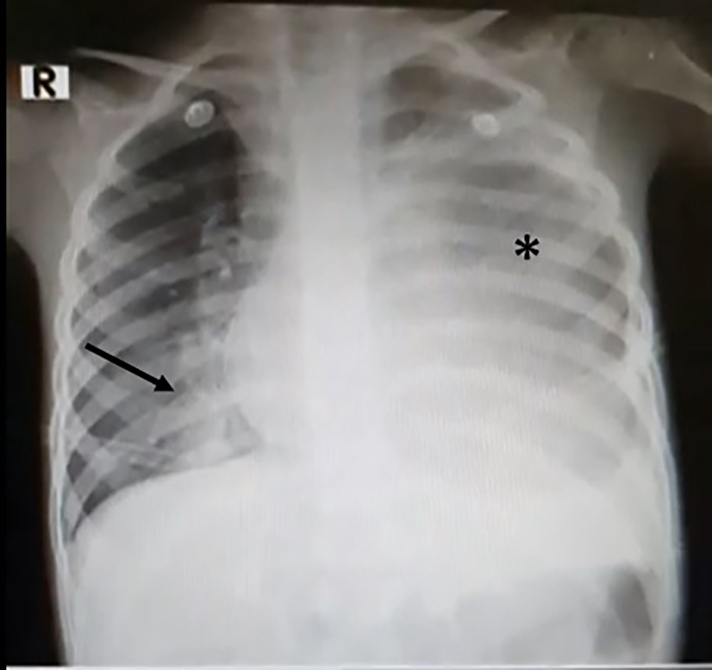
Chest radiograph of five-year-old patient obtained in the emergency department. The left hemithorax is radiopaque, concerning for a possible large pleural effusion (asterisk). There is a normal cardiac silhouette and hazy interstitial opacities concerning for an infectious process present in the right hemithorax (arrow).
